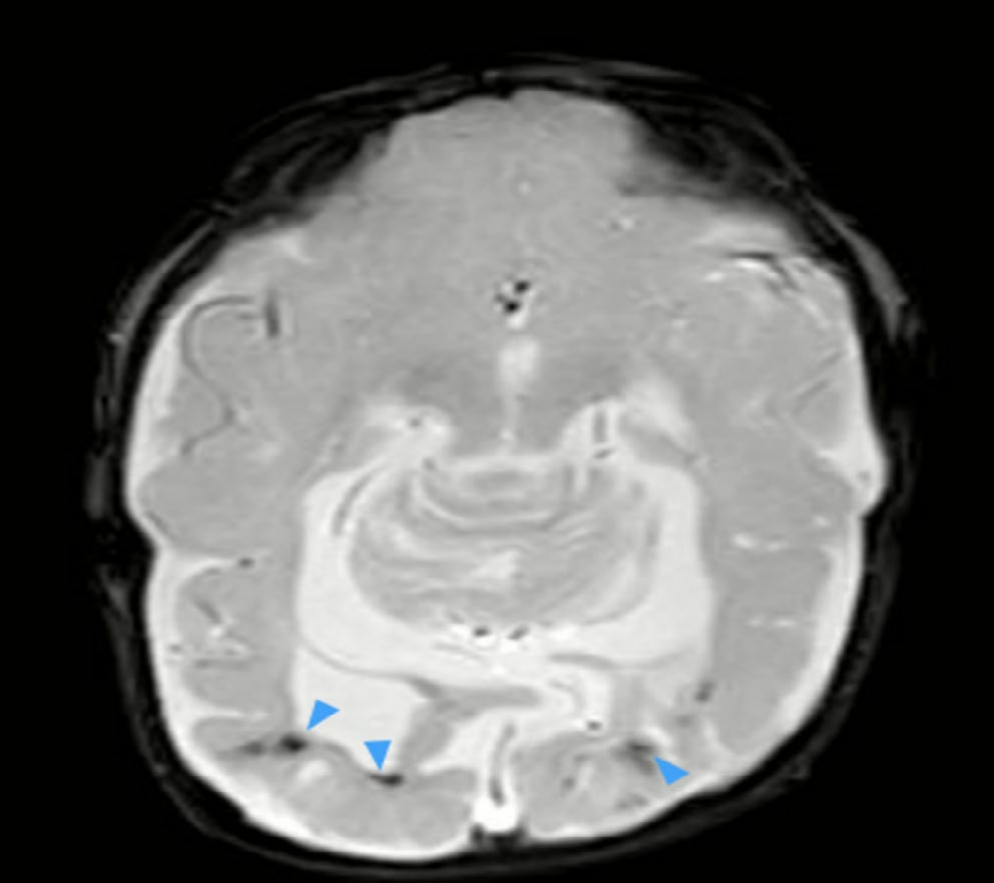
Axial SWI (T2‐weighted*) MRI image of the brain demonstrates a few intra‐ and extraventricular blooming artifacts (blue arrowheads), consistent with blood products.
